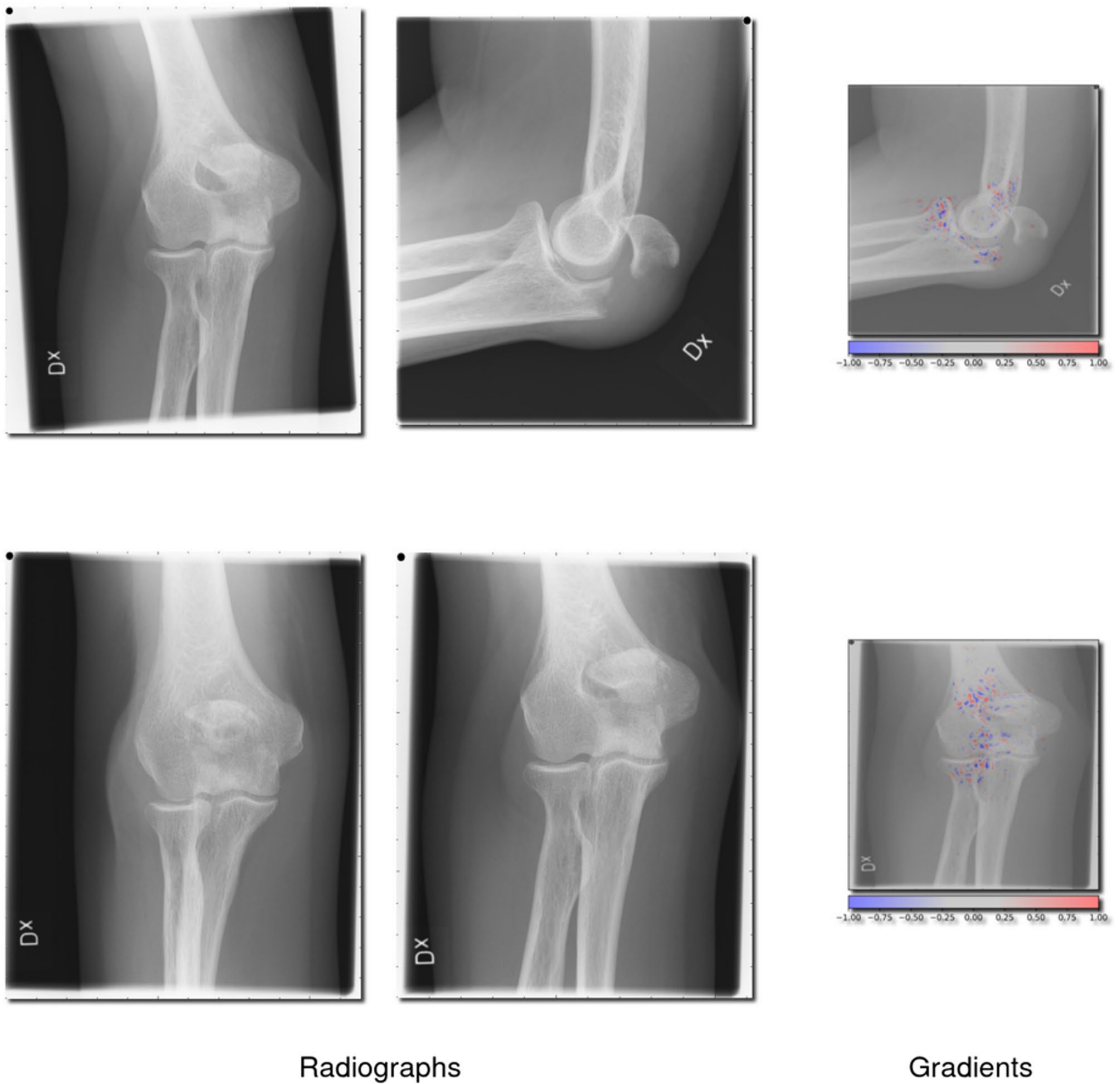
Correctly classified olecranon fractures (2U1B1). This figure showcases 4 radiographs where the network correctly identified a 2U1B1 fracture as being present, as well as 2 radiographs with gradients overlayed showing the network’s interpretation
